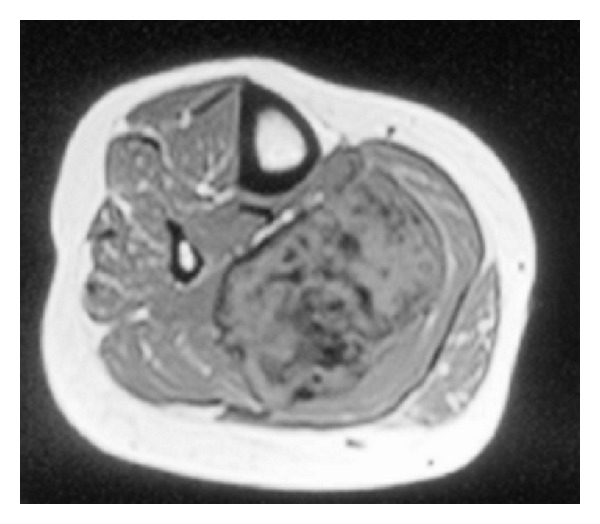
T1-weighted axial MRI of right lower leg showing heterogenous mass.
